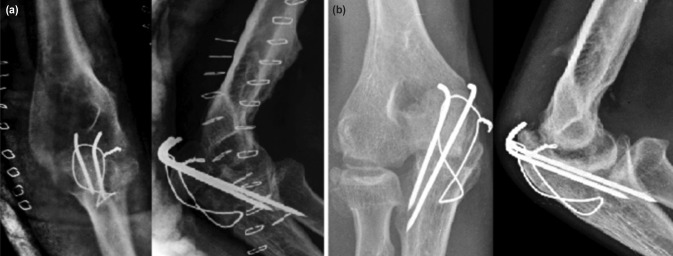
Radiographs (a) After operation. (b) At the end of one year showing arthritic changes.
